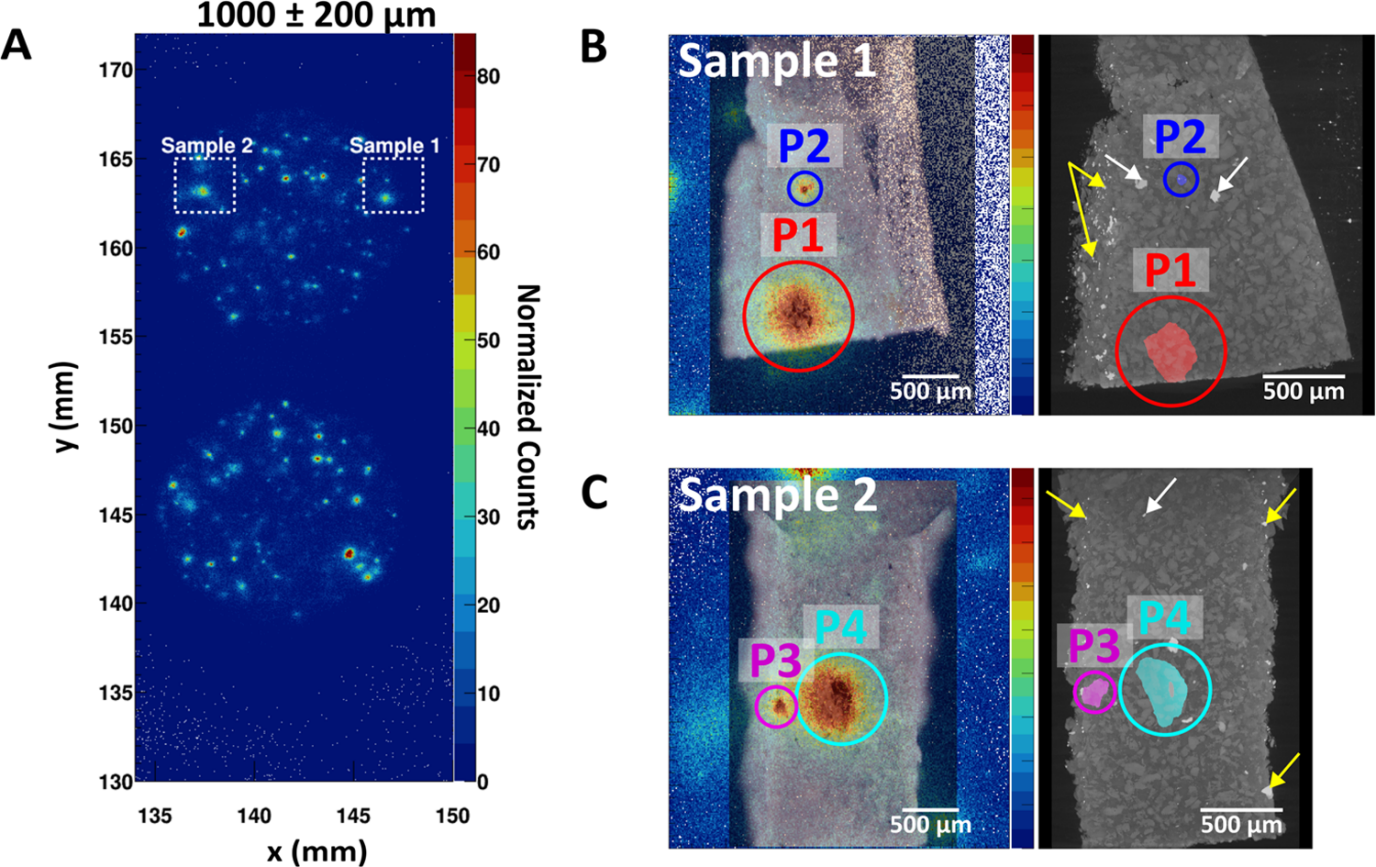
X-ray CT data for 2 regions of interest. (A) Positions of the regions
